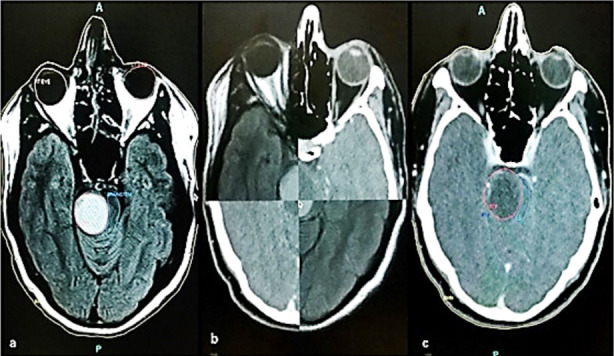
(a): Contouring of GTV and remaining brainstem on MR images. (b): Fusion of MRI & CT images. (c): After fusion, contouring shifted to CT images for planning.
